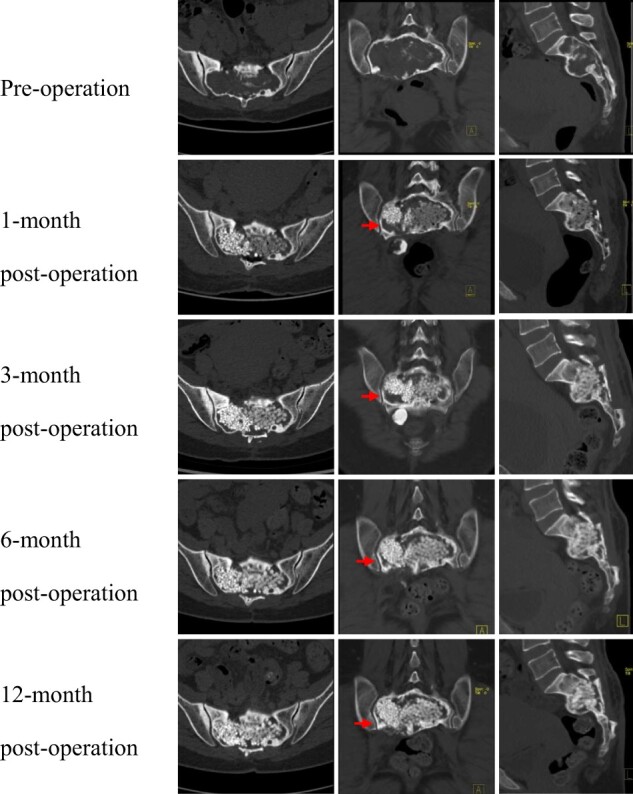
The CT scan of sacrum before and after surgery in Case 1. Arrow, new bone gradually filled the cavity
